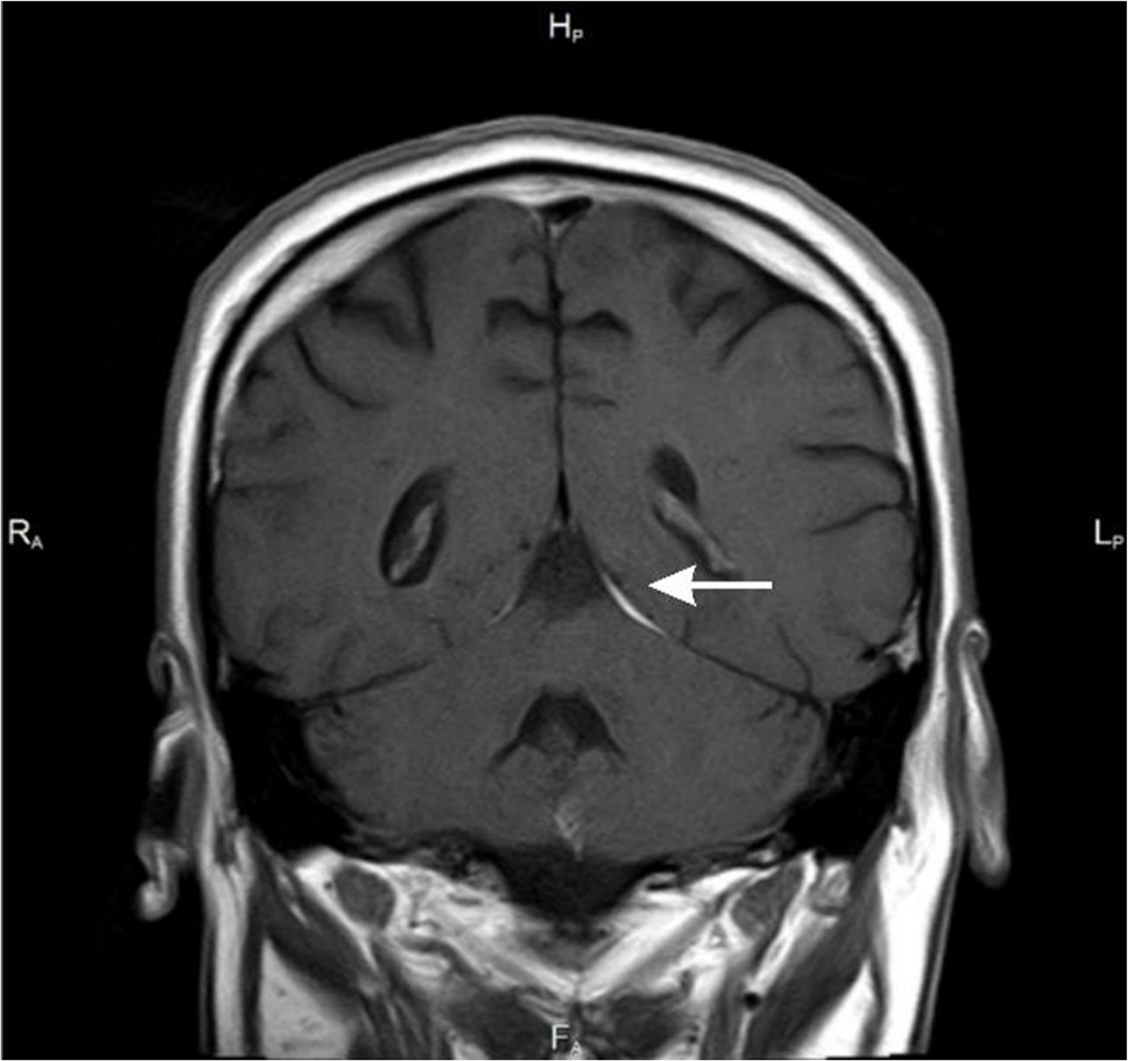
Coronal T1-weighted scan after contrast enhancement - contrast enhancement is also visible in the cerebellar tentorium on the left
